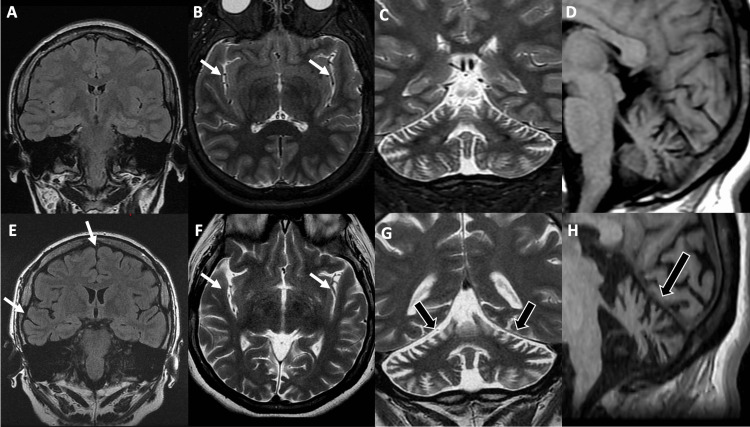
Brain MRI of a female patient with neuronal ceroid lipofuscinosis type 7 at 18 years (A-D superior) and 26 years old (E-H inferior) Comparative coronal fluid-attenuated inversion recovery (FLAIR) (A and E), short-TI inversion recovery (STIR) (B), and T2 (F) images show mild supratentorial volume loss with mild cortical atrophy (white arrows). Comparative coronal T2 (C and G) and T1 (D and H) show prominent folia of the cerebellar hemispheres, representing the progression of cerebellar atrophy (black arrows).
